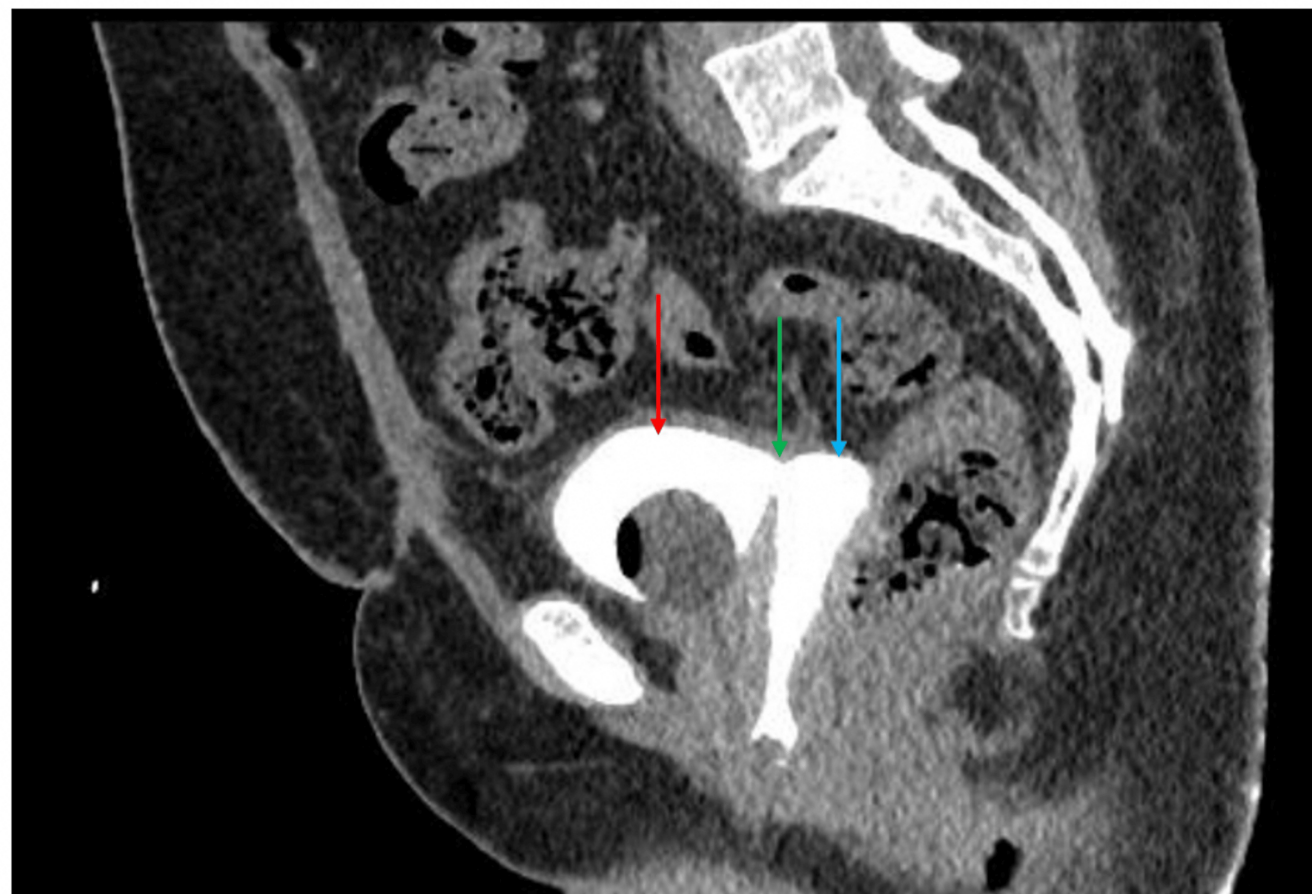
Preoperative CT cystogram with contrast. The red arrow is pointing at the bladder. The green arrow is pointing at the fistula. The blue arrow is pointing at the vagina.
